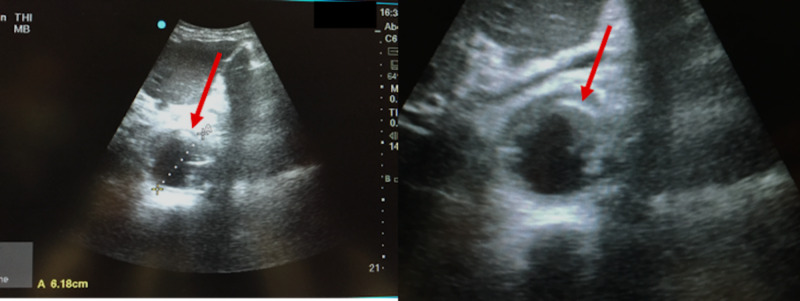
Bedside ultrasonography demonstrating aortic dilation of 6.2 cm with no free fluid in abdomen.
